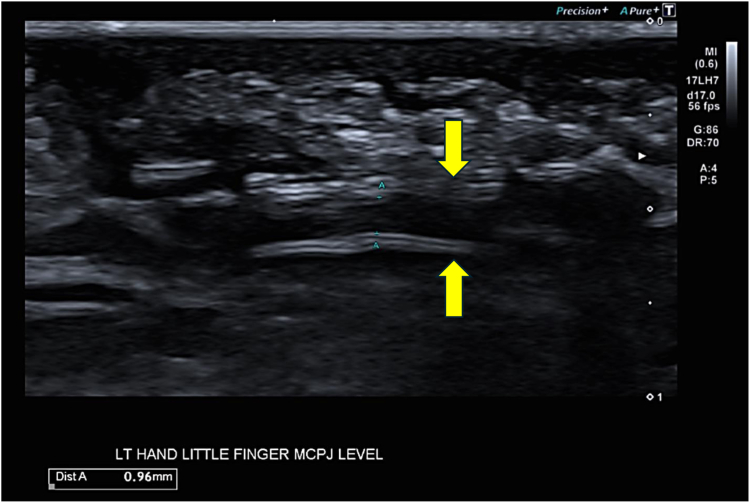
Ultrasound image of the coaptation site at 1 year. Coaptation at the level of the MP joint. Arrows denote the nerve-chamber, and the nerve can be seen in continuity within the chamber.
